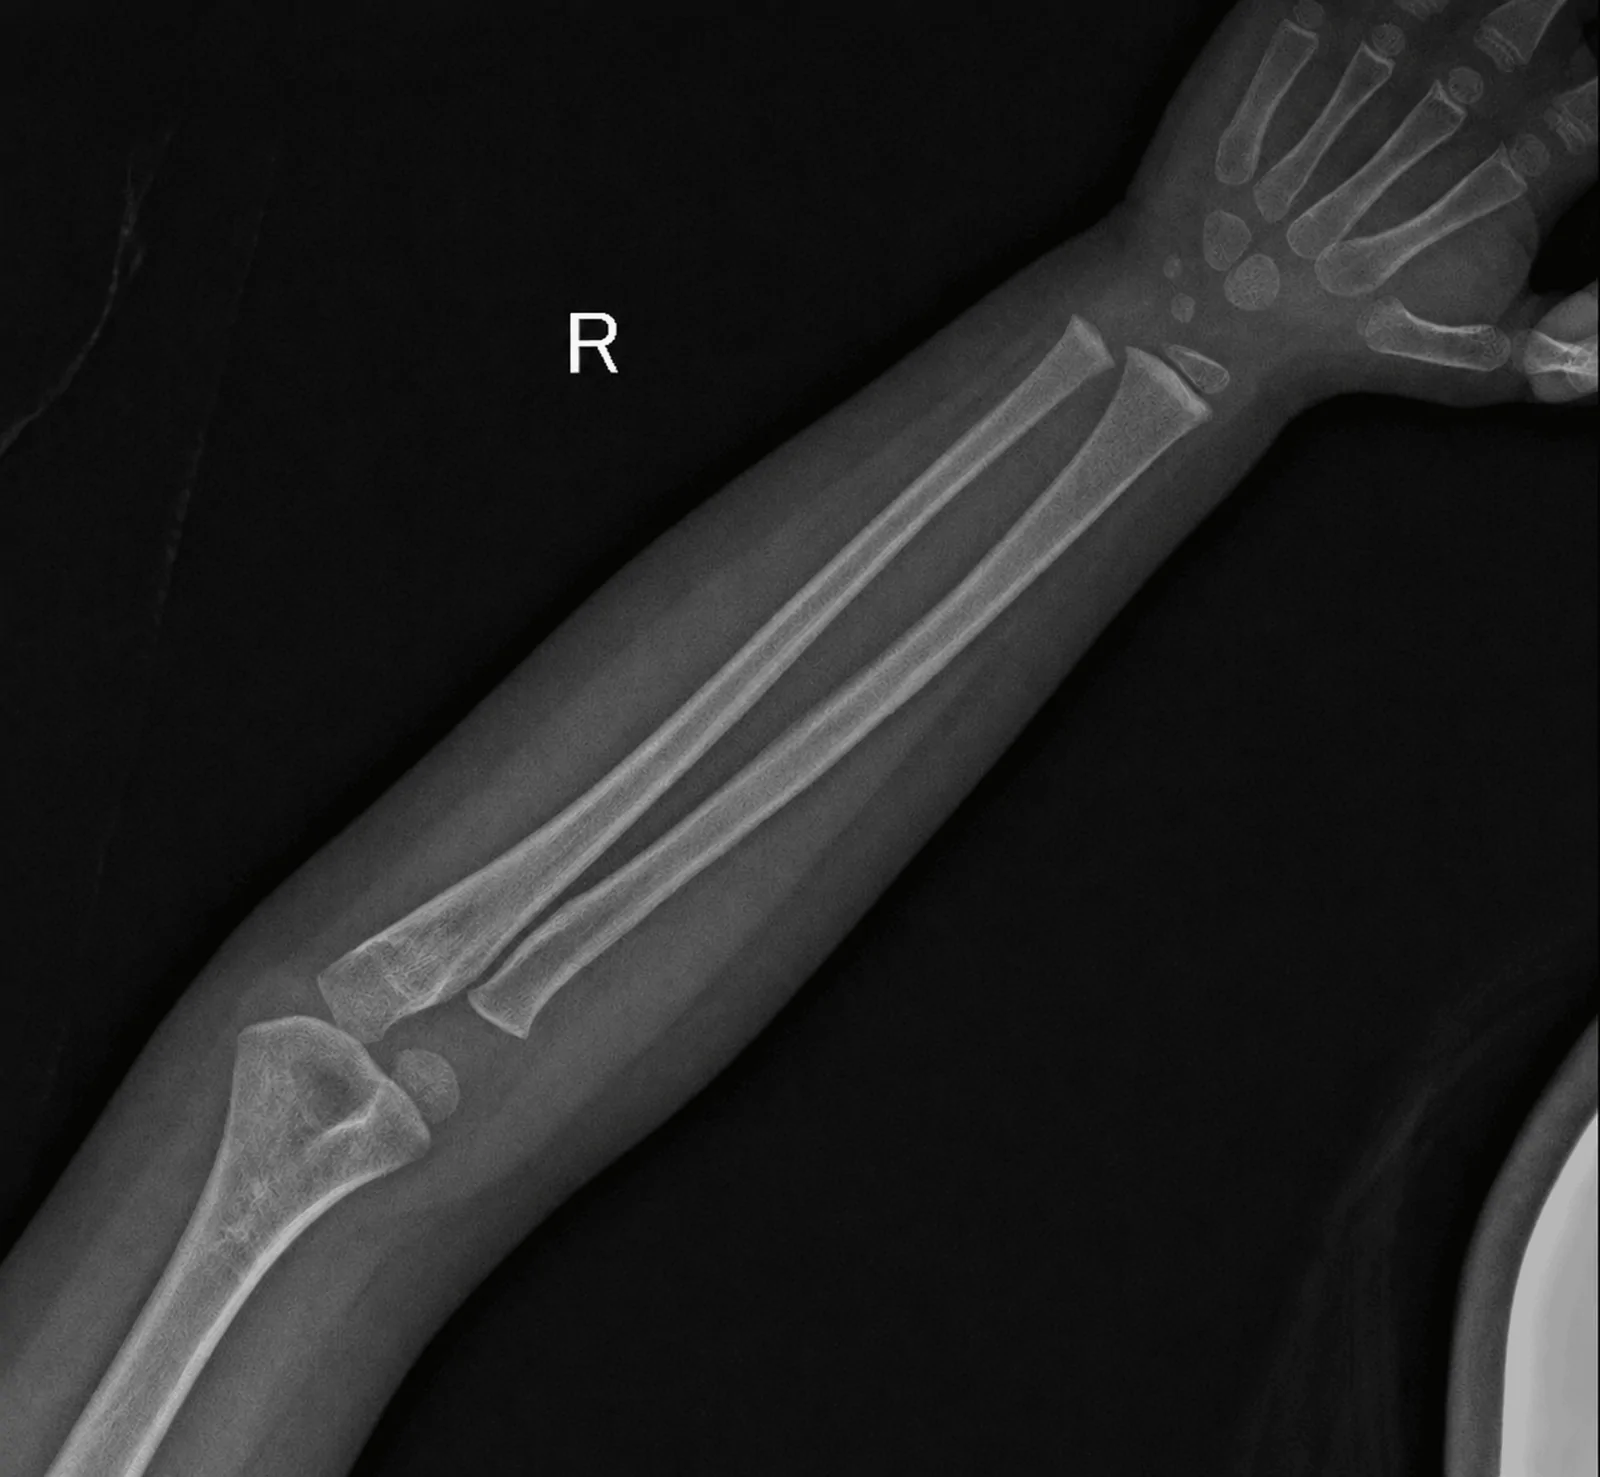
Anteroposterior Radiograph of the Right Forearm The right forearm, including the elbow and wrist, demonstrates no acute fracture or dislocation, with preserved osseous alignment.
